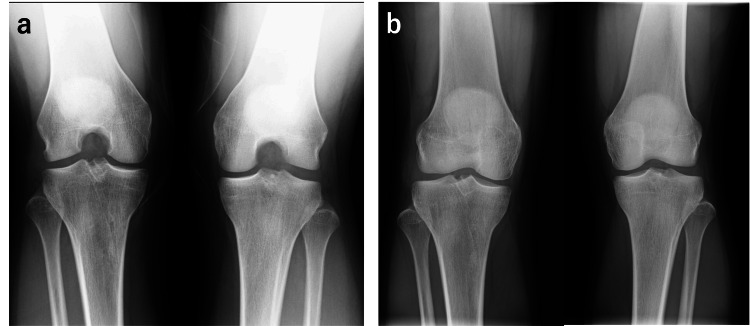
Radiographic findings two years after tendon transplantation (a) Rosenberg view, (b) standing anteroposterior view
